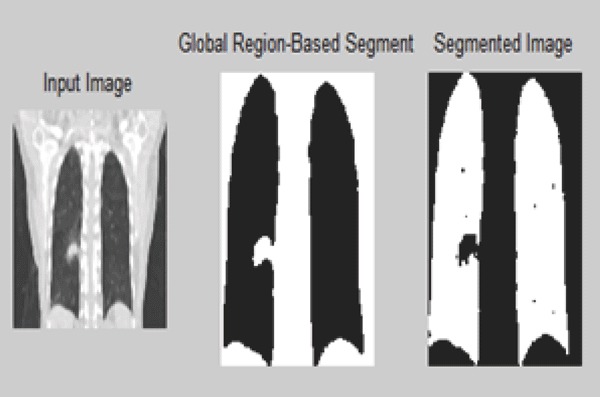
Segmented 4-D CT image using Region Growing.
